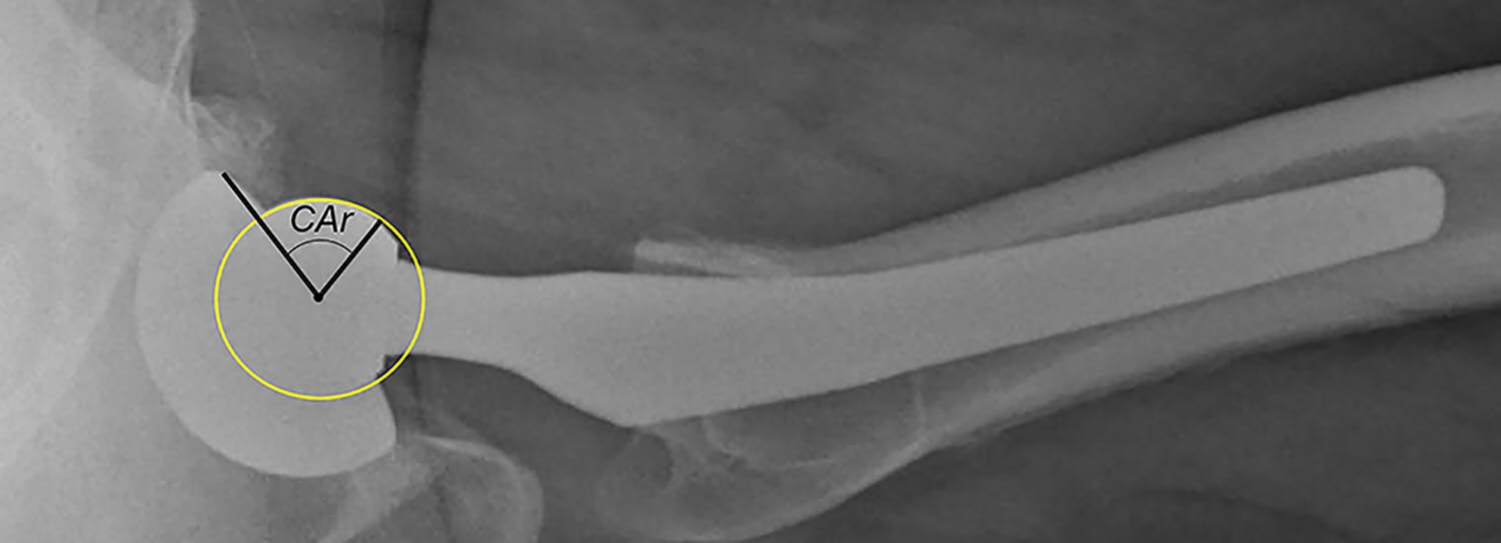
Cross-table lateral radiograph of a left hip demonstrating the method used to measure the radiographic combined anteversion of the components (*CAr*). A best-fit circle was applied to the femoral head. *CAr* was defined as the angle between the line connecting the center of the femoral head (best-fitted circle) to the most anterior rim of the acetabular cup and the line connecting the center of the femoral head to the base of the femoral head, defined as the point where the head loses its sphericity
